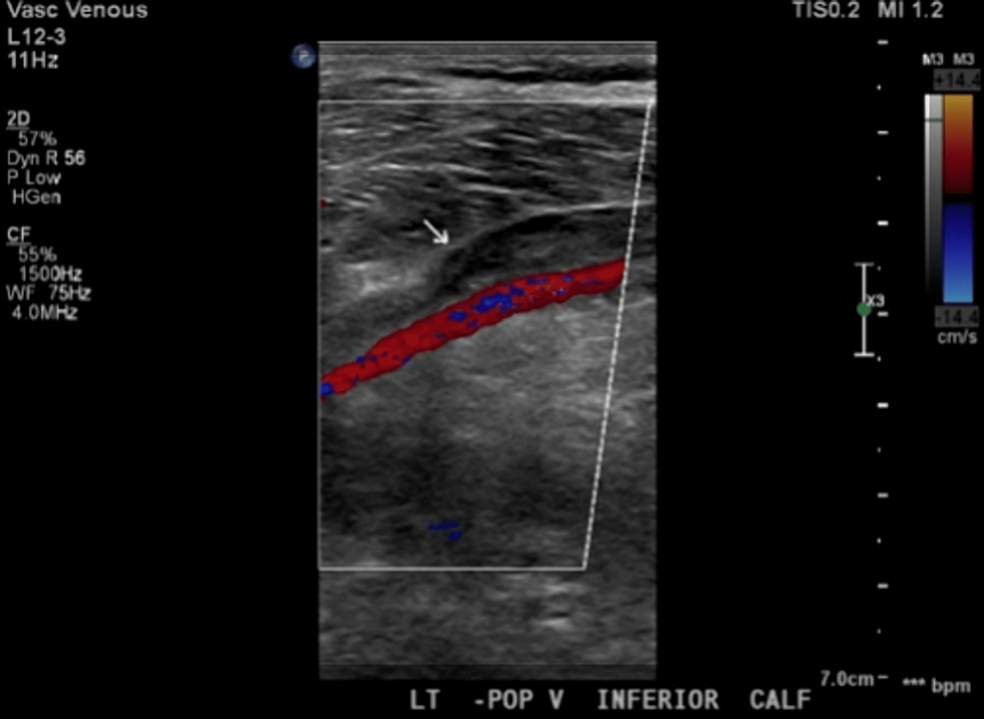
Doppler Ultrasound of the Left Lower Extremity
